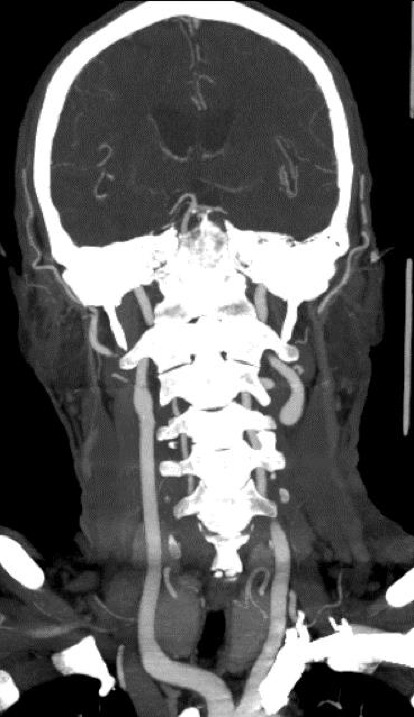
Coronal computed tomography angiography demonstrating normal extracranial carotid arteries.
